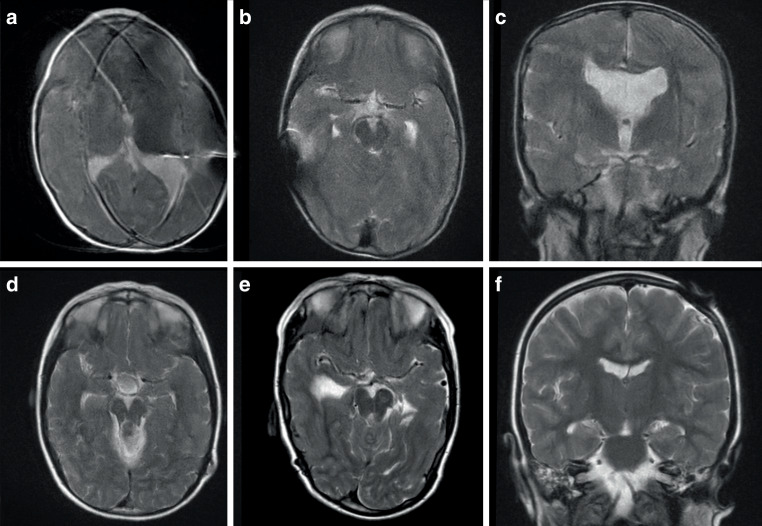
Exemplary images from one reader across different grades of the applied Likert-scale. Transversal T2-weighted image, rated as image quality grade 1 and diagnostic confidence grade 2; transversal T2-weighted image, rated as image quality grade 2 and diagnostic confidence grade 3, coronal T2-weighted image, rated as image quality grade 3 and diagnostic confidence grade 3, transversal T2-weighted image, rated as image quality grade 3 and diagnostic confidence grade 4, transversal T2-weighted image, rated as image quality grade 4 and diagnostic confidence grade 5, coronal T2-weighted image, rated as image quality grade 5 and diagnostic confidence grade 5
